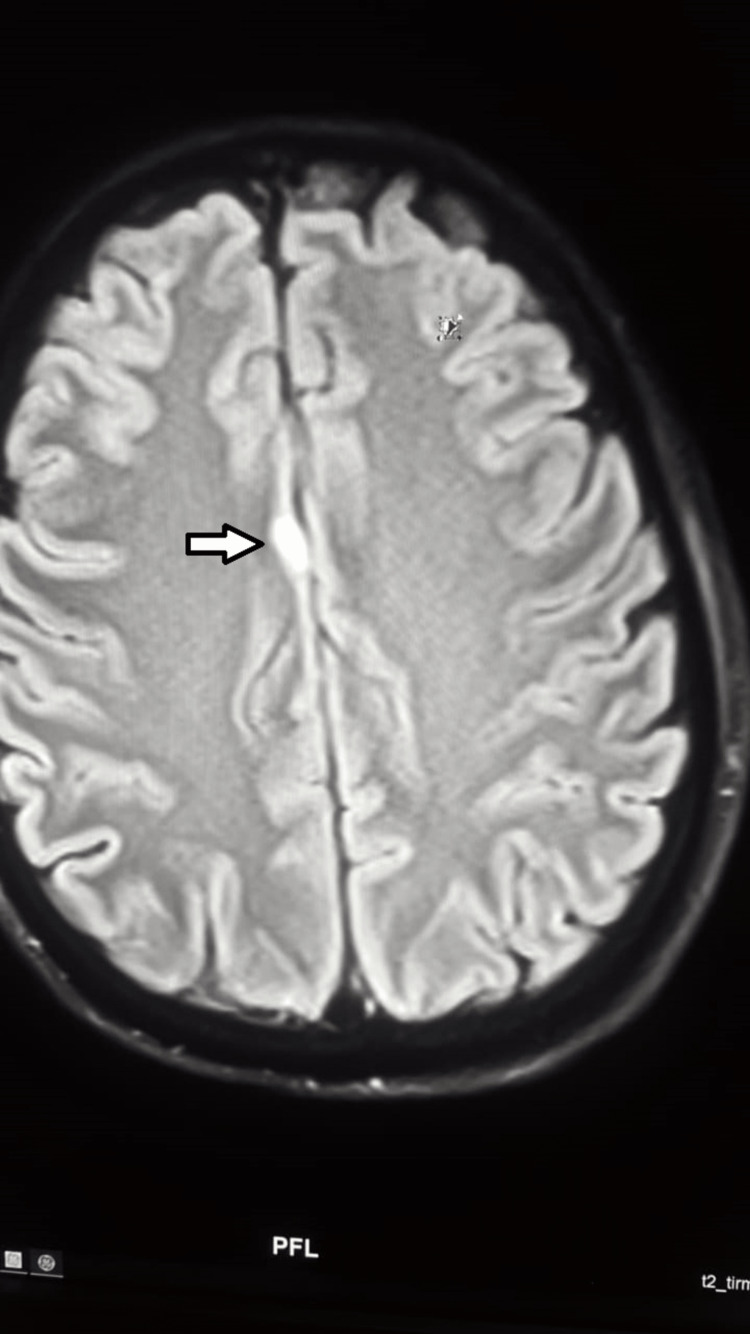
A cortical fluid-attenuated inversion recovery (FLAIR) image A hyperintense lesion in the medial side of the right frontal lobe with restricted diffusion in the diffusion-weighted imaging (DWI), representing a small acute infarction (white arrow).
